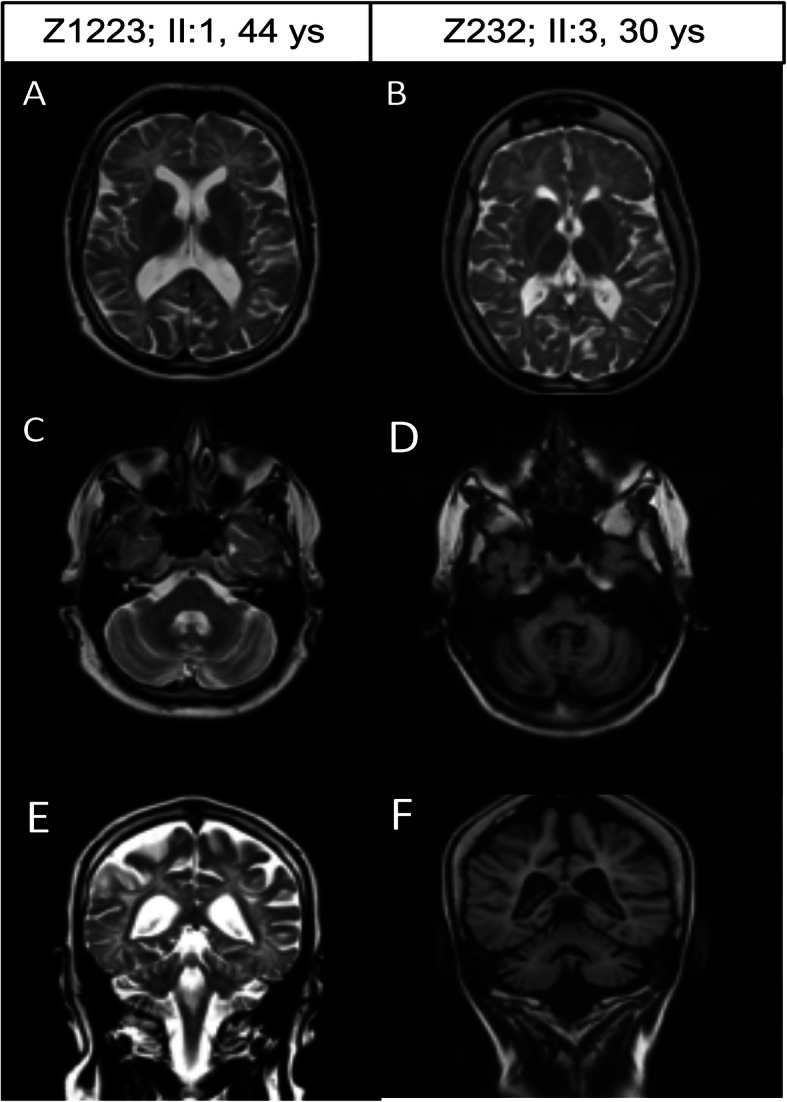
MRI of proband siblings. Axial and Coronal T2 are shown for male proband, Z1223 (on the left, images a, c, e) at the age of 44 years old, and the female proband, Z232 (on the right, images b, d, f) at the age of 32 years old. Axial T2 weighted MRI of brain at the level of basal ganglia (A and B: Turbo Spin Echo). Images show in male proband an evident dilatation of the supratentorial ventricular system, diffused hyperintensity of the white matter mainly in the frontal site (a). The female proband possesses the same pattern, but diffused hyperintensity of the white matter is present in both frontal and posterior lobe. A marked hyperostosis is evident (b). Axial T2 weighted MRI of brain at the level of posterior cranial fossa (C and D: T2 FLAIR). Images show in male proband an hyperintensity of the middle cerebellar peduncle and dilatation of the fourth ventricle of the prepontine cistern and subarachnoid spaces (c). The female proband possesses the same pattern although each alteration is more severe (atrophy of cerebellar peduncles, enlargement of the fourth ventricle pre-pontine cistern and subarachnoid spaces) (d). Coronal T2 weighted (E = T2 Turbo Spin Echo; F = T1 Spin Echo). Both male (e) and female (f) proband show a marked enlargement of cortical subarachnoid spaces, enlargement of middle cell of the lateral ventricle enlargement of the fourth ventricle and widespread hyperintensity of the white matter
